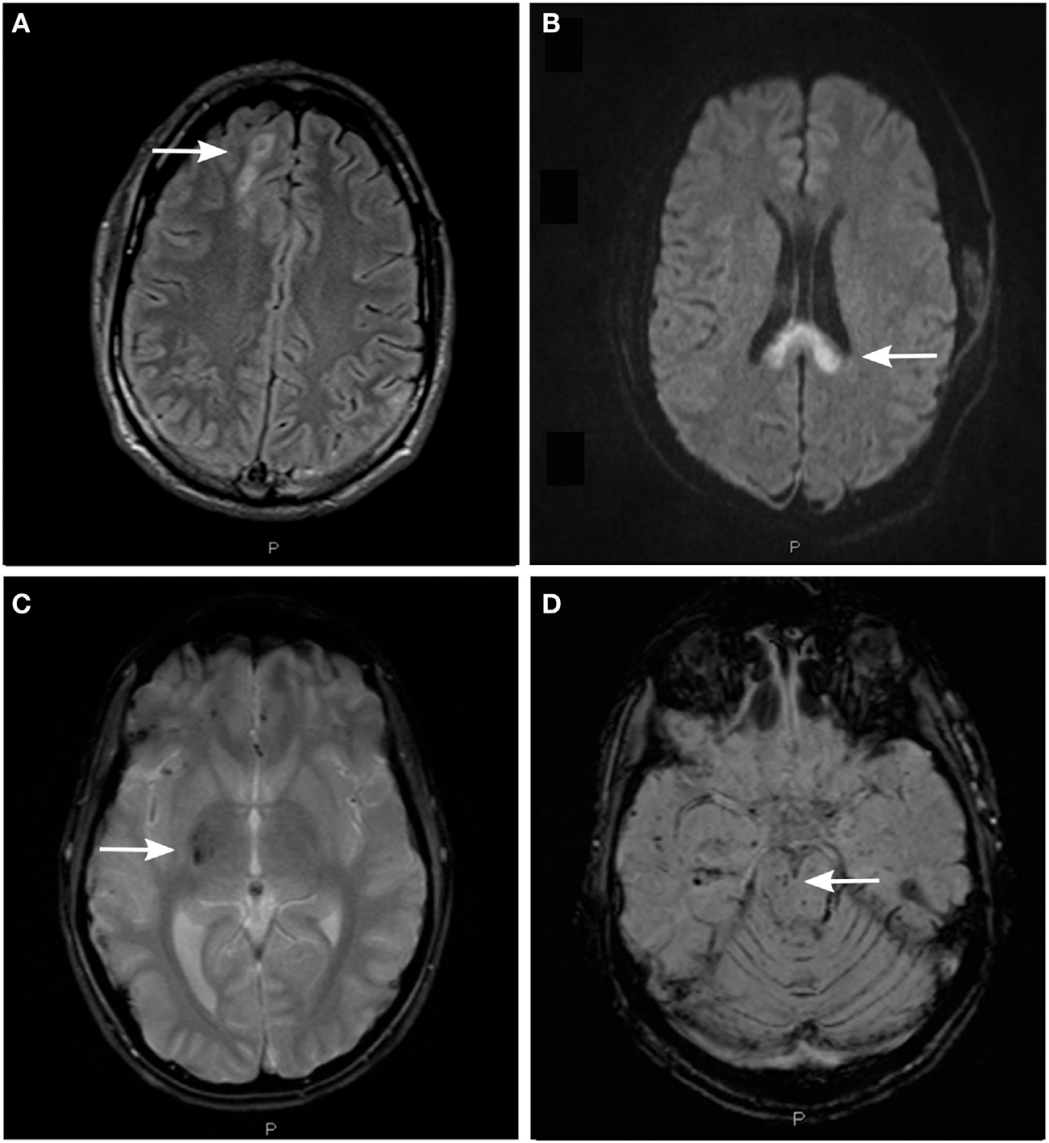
Detection of axonal injury with conventional magnetic resonance imaging (MRI) using different MRI sequences. (A) Fluid-attenuated inversion recovery (FLAIR) image depicting non-hemorrhagic diffuse axonal injury (DAI)-associated lesions in the subcortical white matter of the right cerebral hemisphere (arrow). (B) Diffusion-weighted image (DWI) depicting non-hemorrhagic DAI-associated lesions in the body and splenium of the corpus callosum. (C) T2*-weighted gradient echo (T2*GRE) image depicting hemorrhagic DAI-associated lesions in the right thalamus and putamen (arrow). (D) Susceptibility-weighted image (SWI) depicting hemorrhagic DAI-associated lesions in the right mesencephalon (arrow) and in the white matter of right temporal lobe.
